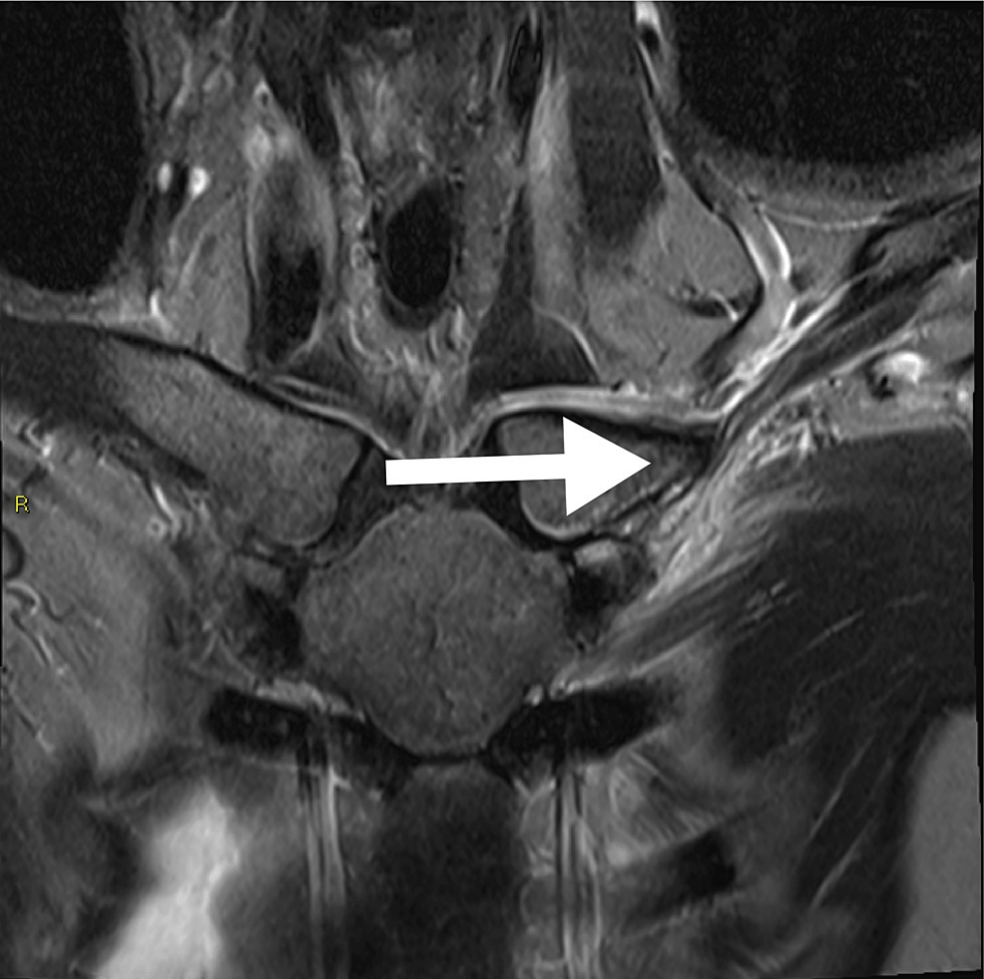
Coronal fat suppressed fluid sensitive MRI shows edema in the left distal first rib and surrounding soft tissue, in keeping with costochondritis. There is also mild signal change in the adjacent medical clavicle head. There is neither significant effusion of the left sternoclavicular joint, nor is there marrow edema in the sternum. The overlying pectoralis major muscle is edematous, likely secondary to reactive change.
